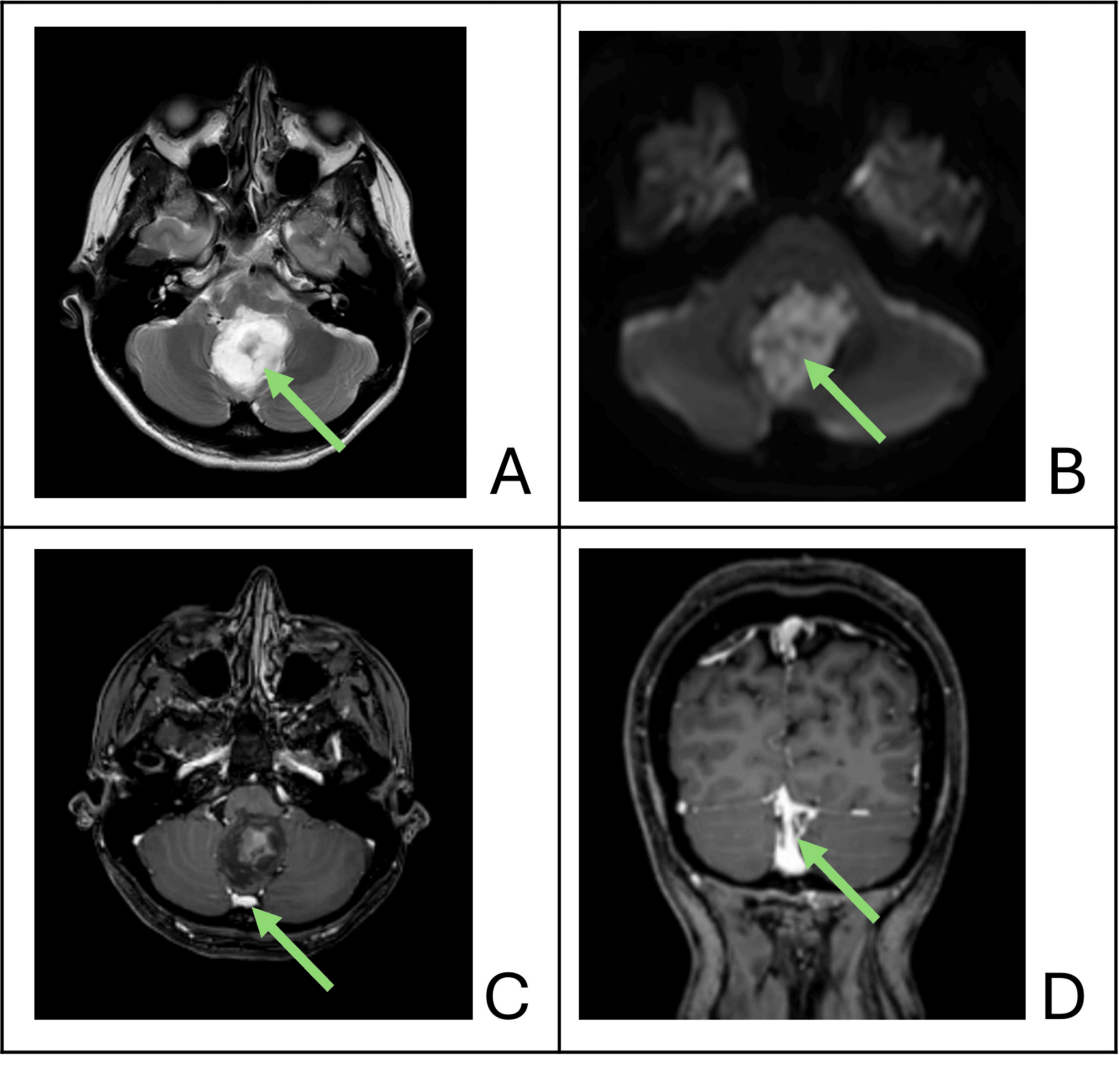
Magnetic resonance imaging of fourth ventricular tumour and dural venous sinuses (A) Axial T2 image showing hyperintense fourth ventricular lesion (arrow) with compression of the brainstem and cerebellar peduncle; (B) Axial diffusion-weighted image showing restricted diffusion (arrow) consistent with epidermoid tumour; (C) Axial T1 post-gadolinium image showing large midline occipital sinus (arrow) and non-enhancing epidermoid tumour; (D) Coronal T1 post-gadolinium image showing dominant occipital sinus (arrow) with bilateral hypoplastic transverse sinuses
